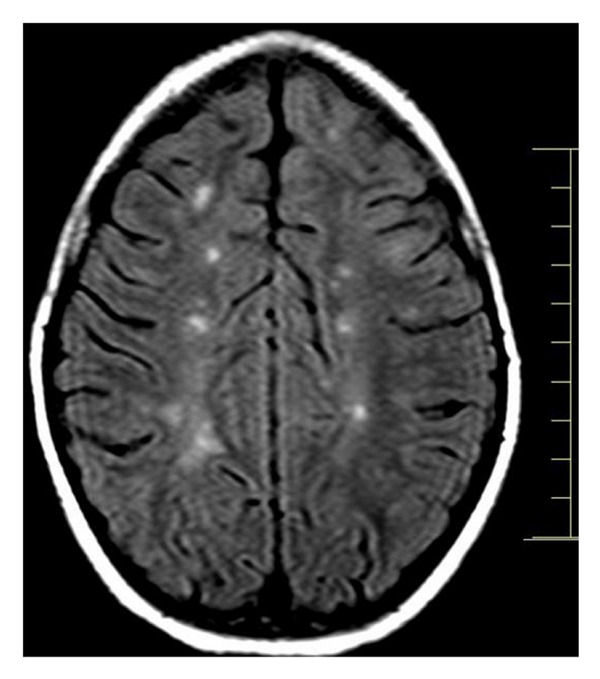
Brain MRI showing multiple juxtacortical lesions.
